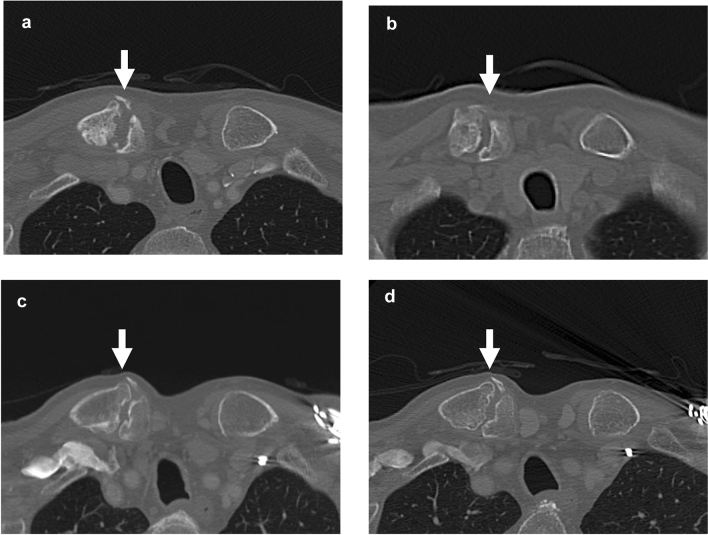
Follow-up contrast-enhanced CT examinations over the next 6 years showed gradual narrowing of the separation between the bone fragments, but no adhesion of the fragments (arrows). a: CT on June 20, 2011; b: CT on May 20, 2013, c: CT on November 8, 2016, d: CT on November 2, 2017
